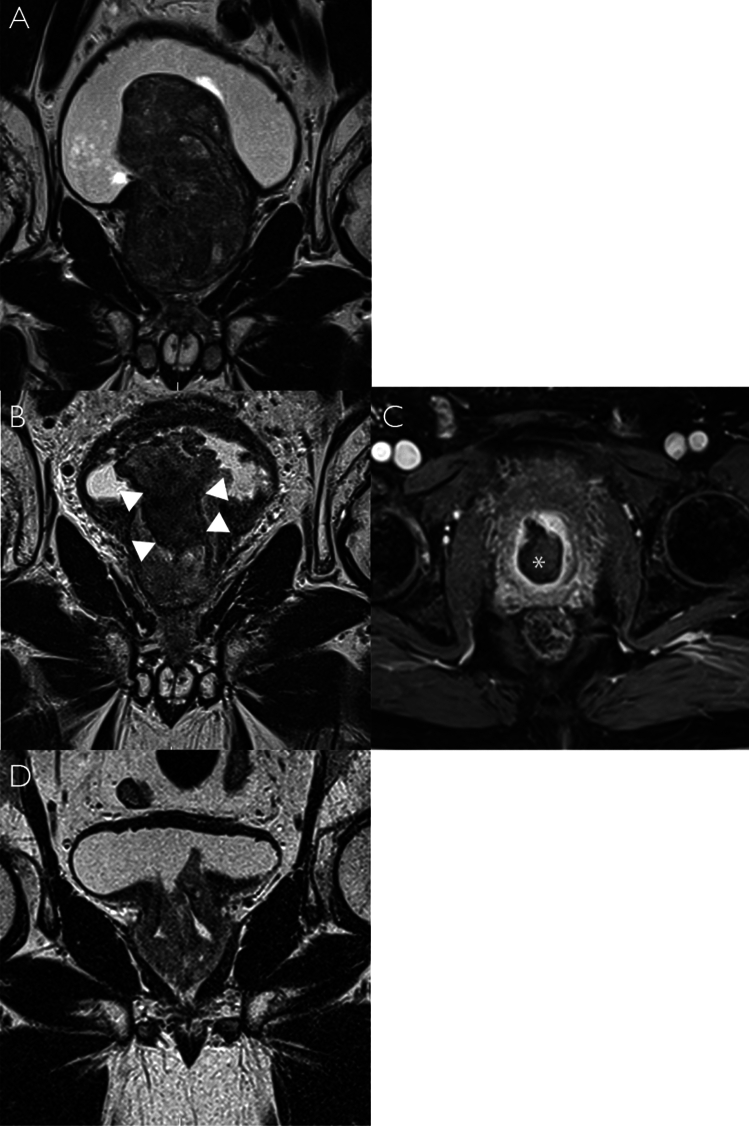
MRI prior to PAE, at 3 months and 12 months post-PAE A Coronal T2 image showing a 165 ml prostate gland with a large median lobe B Coronal T2 image showing the infarcted median lobe within a cavity in the prostate (white arrows) and C Axial post-contrast T1 image showing the non-enhancing sloughed tissue (white asterisk) D Coronal T2 image at 12 months showing significant PV reduction and interval removal of the sloughed median lobe
